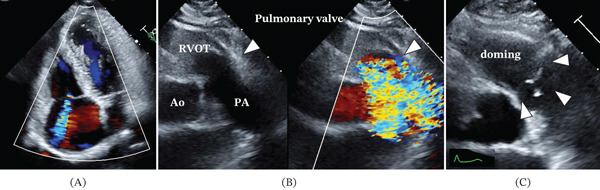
Echocardiographic findings at rest. (A) Apical four‐chamber view showing right atrial enlargement. (B) Parasternal short‐axis view demonstrating turbulent flow across the pulmonary valve. (C) Systolic dome‐shaped morphology of the pulmonary valve consistent with valvular stenosis.
